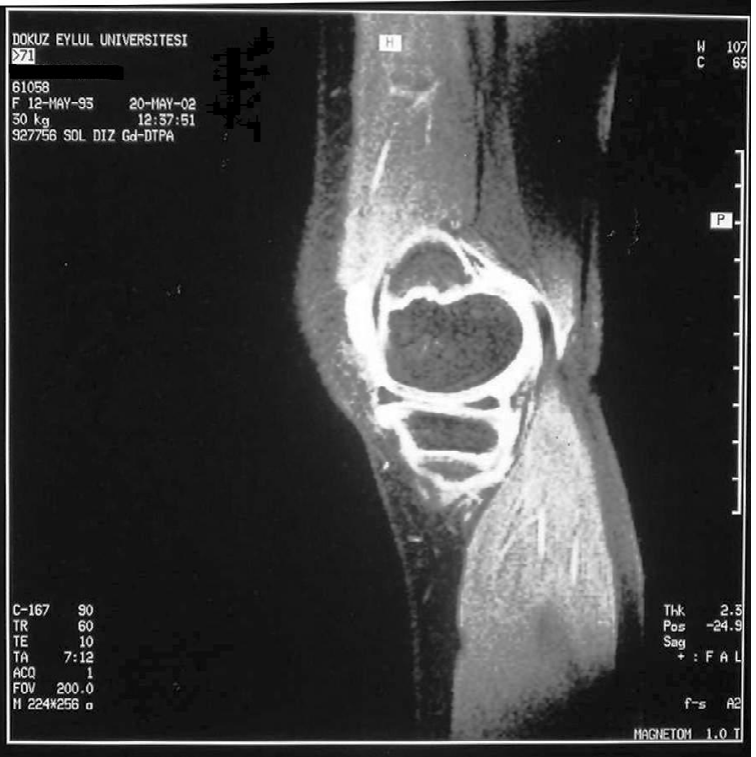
Contrast enhanced MRI of the left knee demonstrating chronic inflammation with synovial thickening.
